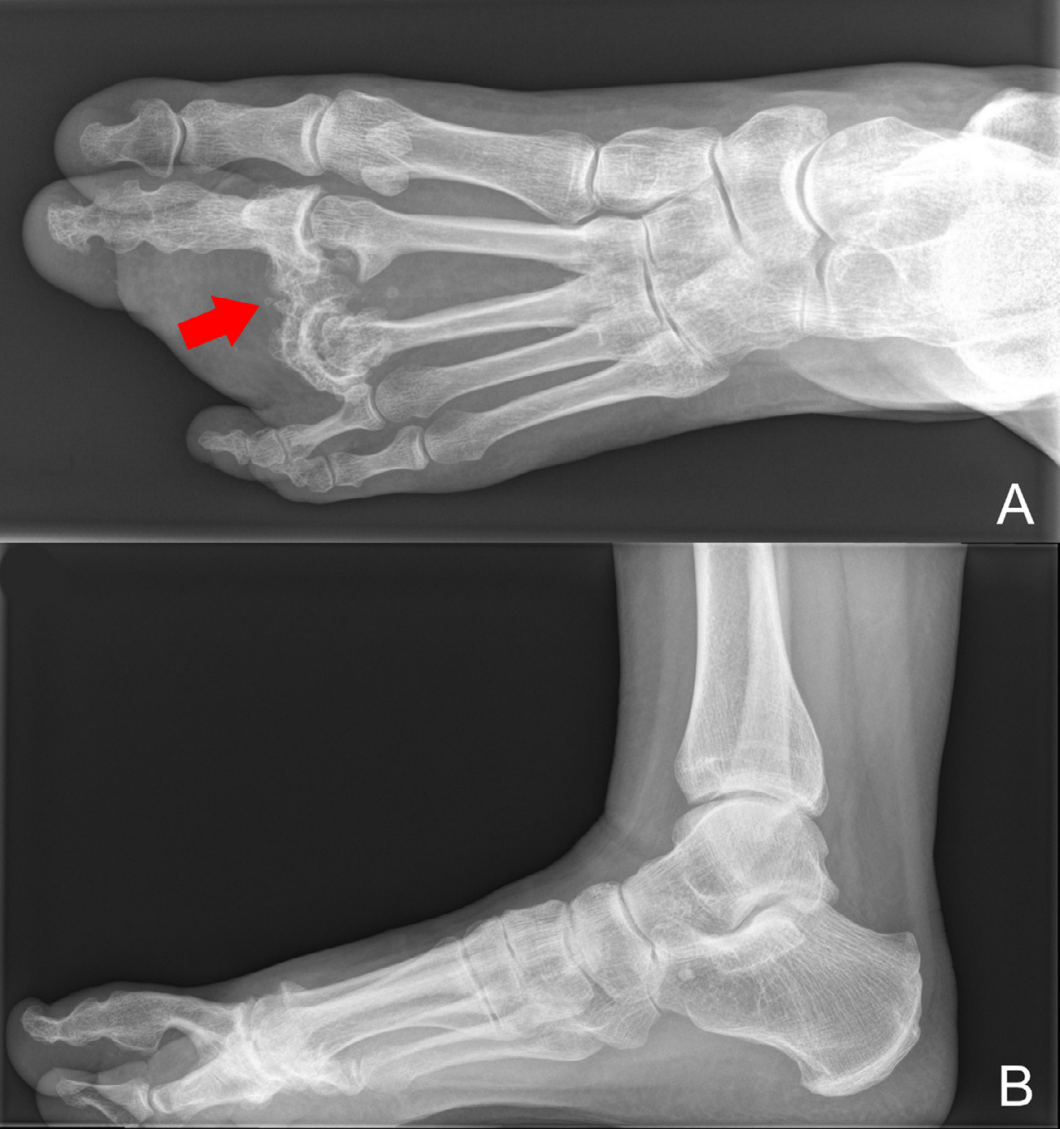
Conventional radiographs of the left foot of case 3. Status after amputation of the third toe through the MTP joint in 1980. 7A: A-P radiograph showing bone exostosis and osteoarthritic deformed bones of the second and third toe (arrow). 7B: Lateral radiograph showing elevation of the second toe because of the plantar swelling.
